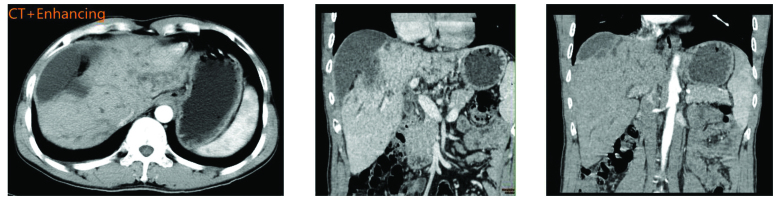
Multiple plane enhanced computed tomography of the upper abdomen revealed no recurrent hepatocellular carcinoma lesion in the liver.
